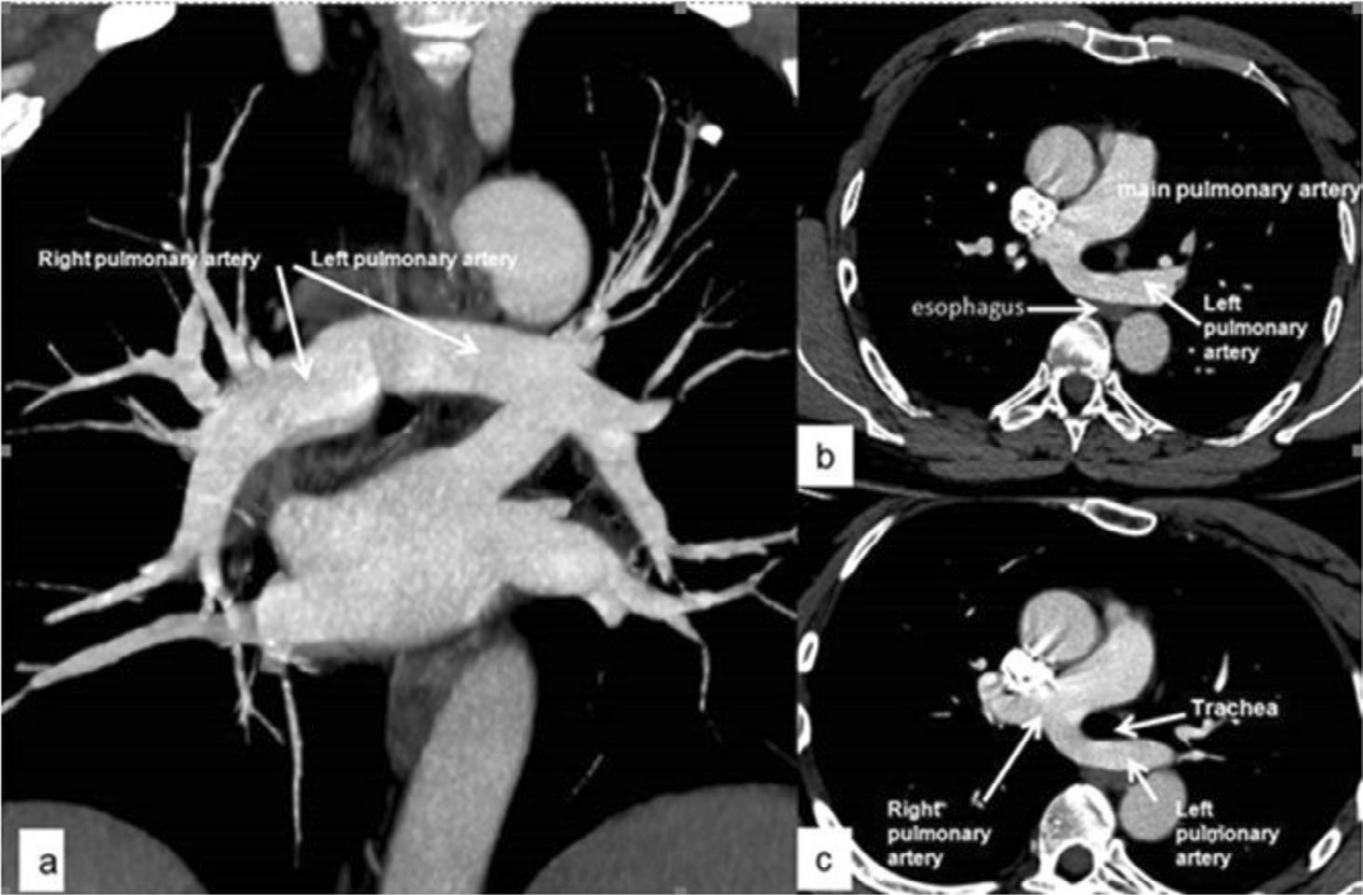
Contrast‐enhanced thoracic computed tomographic scan in the mediastinal window, coronal (a) and axial (b, c) planes, demonstrates that the left main pulmonary artery originates from the right pulmonary artery and courses posterior to the trachea and anterior to the esophagus before reaching the left lung hilum, consistent with a pulmonary artery sling.
